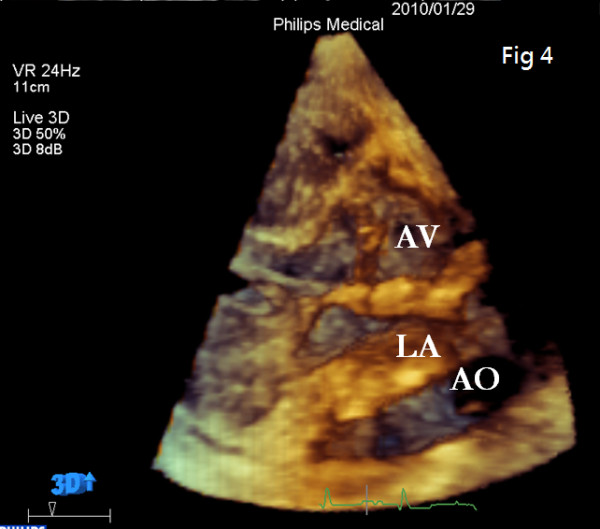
Three-dimensional echocardiography view: LA is constrained between the aortic valve (AV) and a luminal structure with pulsatile character is suggestive of the aorta.
